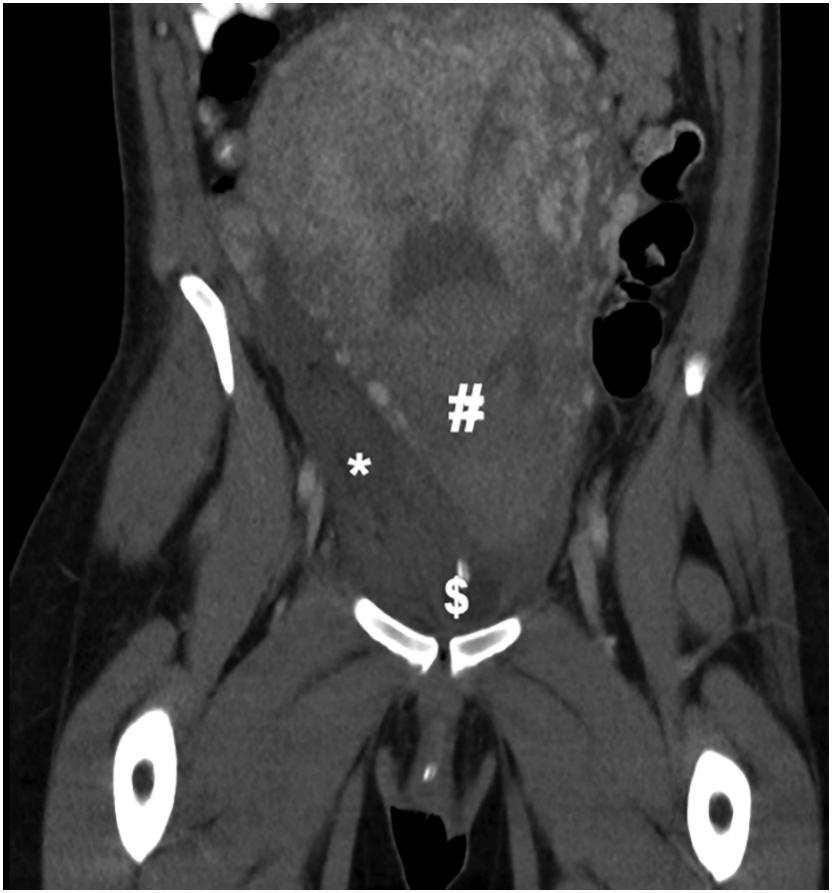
CT scan showing the right broad ligament haematoma*, blood products in the uterine cavity#, and indwelling urinary catheter balloon$.
